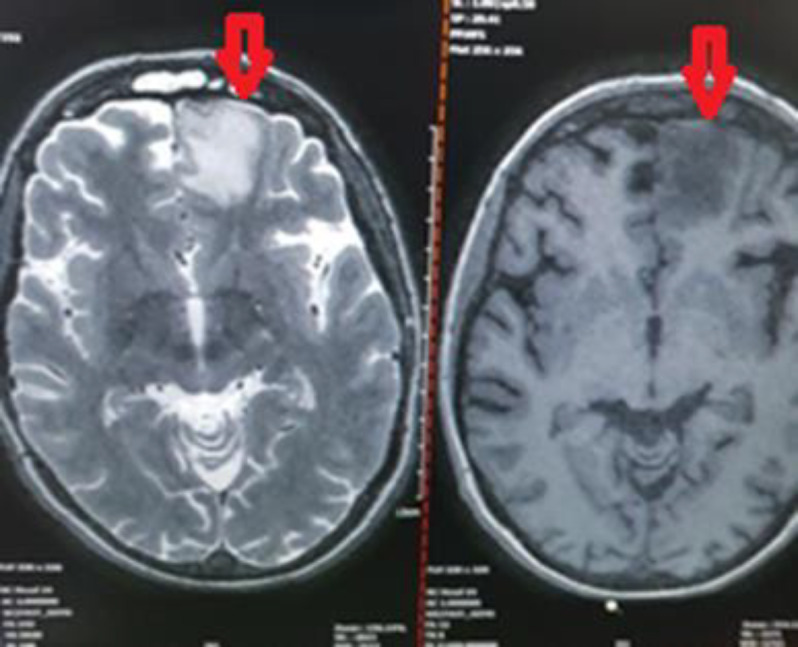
The brain MRI image (MRI-T2 flair view) of an intracranial hyper-intense lesion with dimensions of 20×20 mm in the left frontal lobe (Red arrow)
